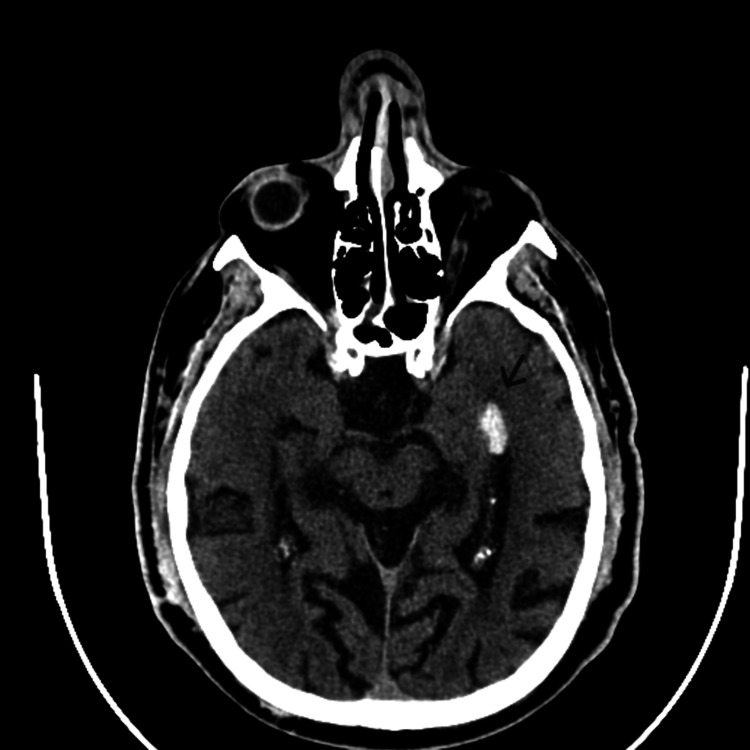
Non-contrast-enhanced brain CT showing a well-defined and rounded acute hyperdensity in the temporal horn of the left lateral ventricle (arrow)
